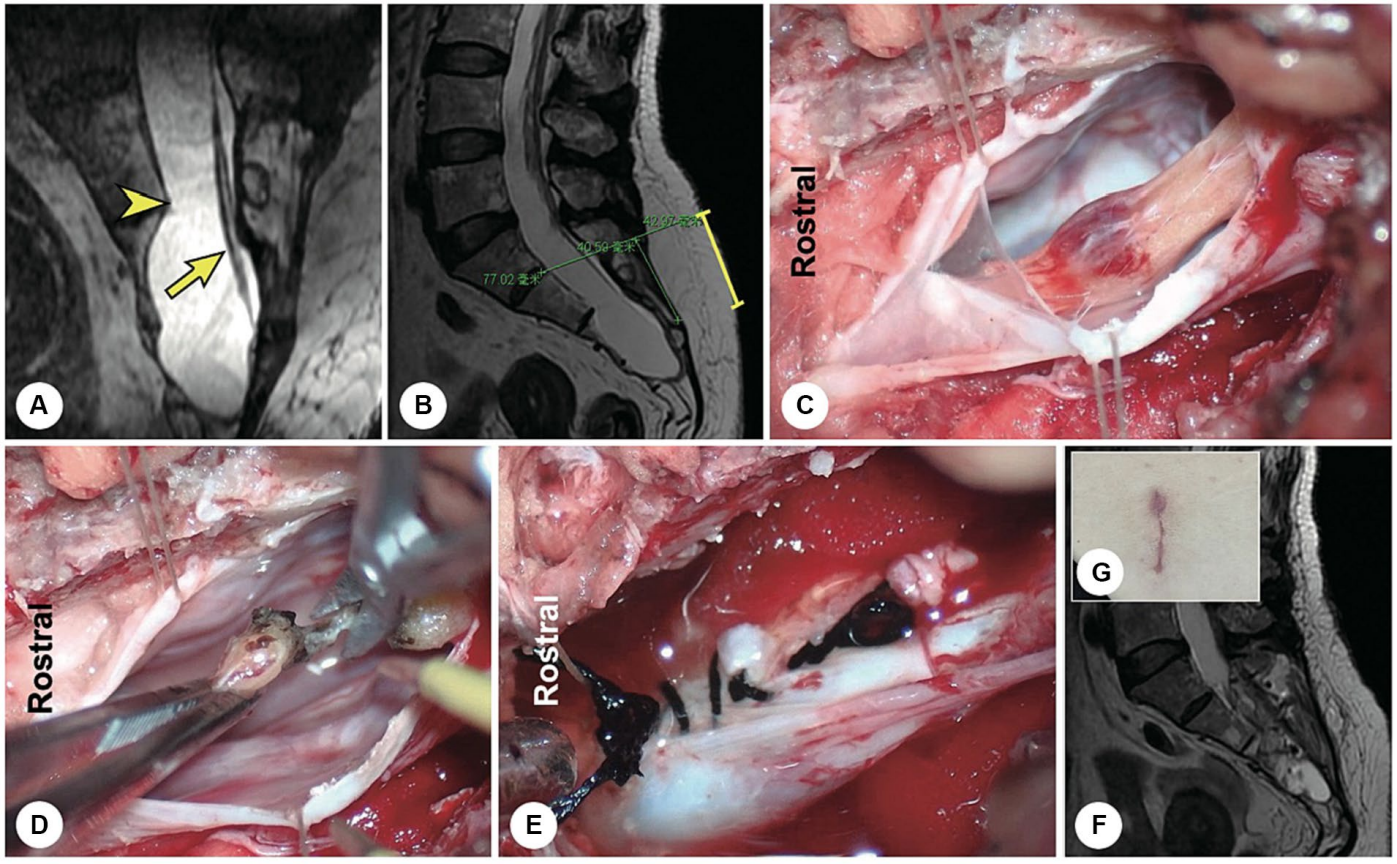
A representative patient with a filum terminale cyst. (A) Preoperatively, reconstructed spinal MR image showing a sacral cyst at the S2-4 level with a thickened filum terminale and wide leakage neck (the arrowhead indicates the cystic leakage orificium, and the arrow indicates the filum terminale). (B) A 4 cm precise minimally invasive incision was made (yellow line segment). (C) Intraoperatively, the filum terminale was found within the cyst cavity. (D) The filum terminale was cut off after electric coagulation. (E) The leakage orificium was ligated. (F) Follow-up MRI showed that the cyst had disappeared and that there was no recurrence. (G) The 4 cm long skin incision healed well.
